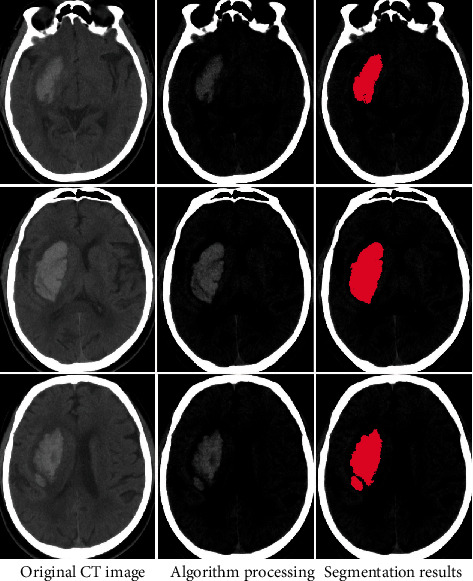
The CT images were preprocessed by the CNN algorithm.
